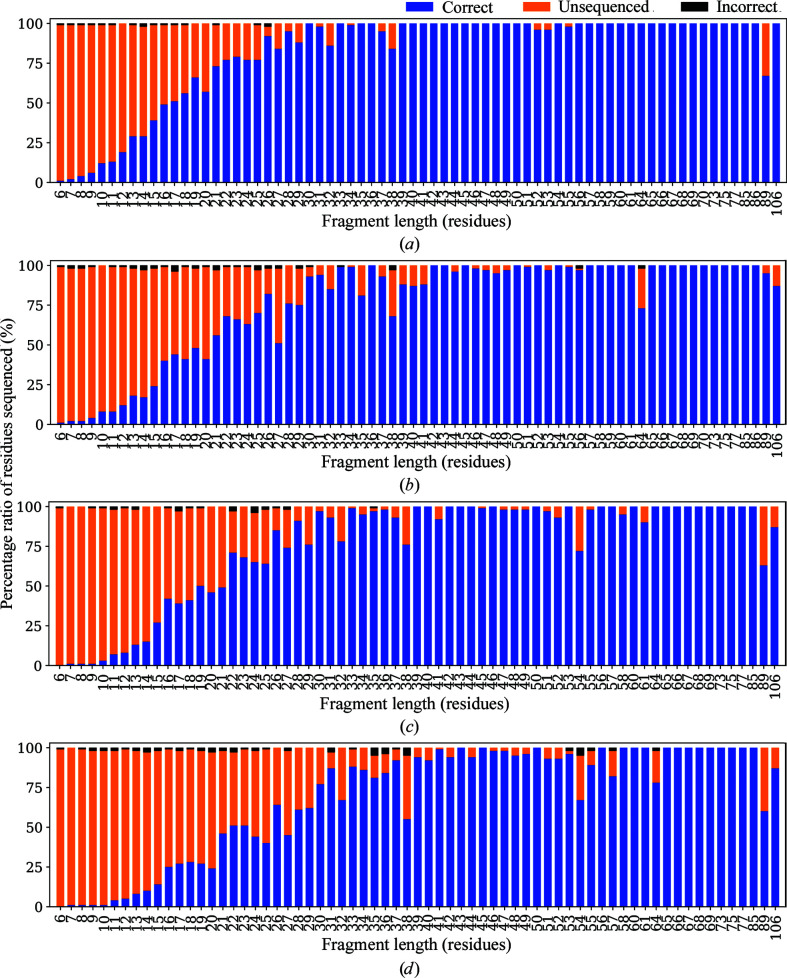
Average success rates in sequencing fragments of different lengths for 109 models using two different chain traces and reference structures. (*a*) Fragments cut from the deposited models sequenced using an EM reference structure. (*b*) Fragments cut from the deposited models sequenced using an X-ray reference structure. (*c*) Fragments from the *Buccaneer* models sequenced using an EM reference structure. (*d*) Fragments from the *Buccaneer* models sequenced using an X-ray reference structure.
